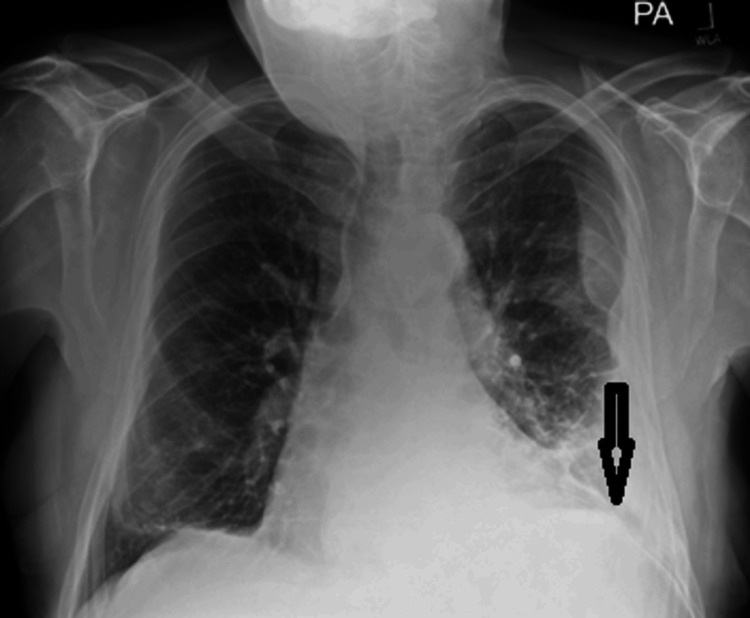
Post drainage posteroanterior view chest X-ray showing partial resolution of empyema, chest drain in-situ and left lower zone pulmonary infiltrates
